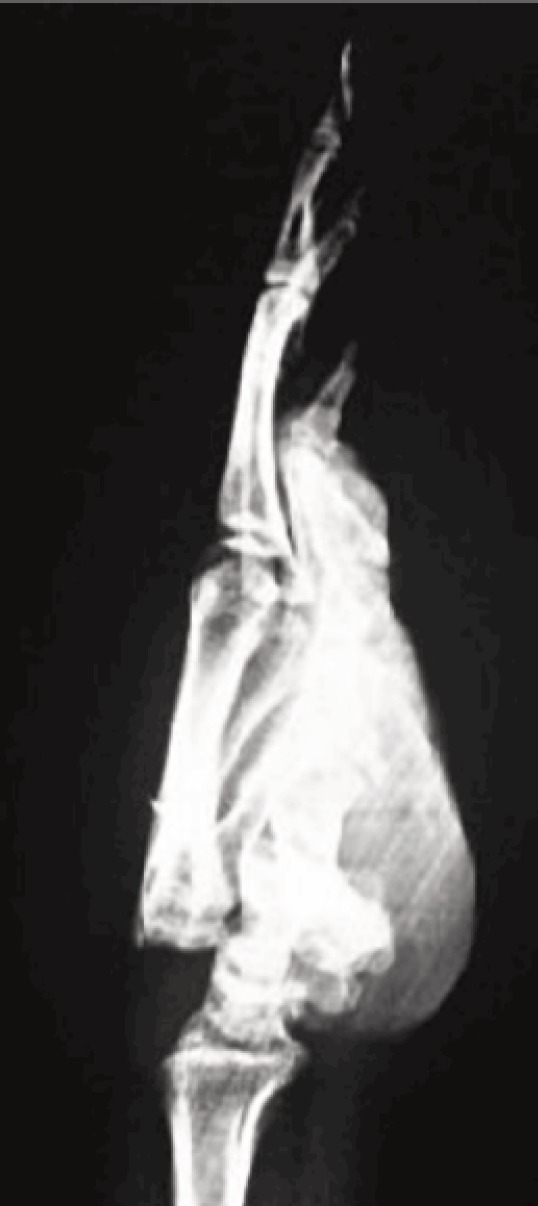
Lateral radiograph view showing Classification Type A. Dorsal dislocation of multiple metacarpals without any associated fractures.
